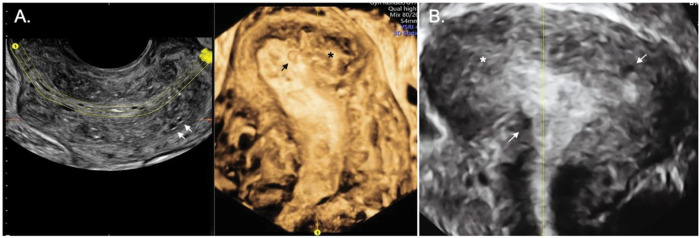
Two and three-dimensional ultrasound imaging of a uterus with diffuse type adenomyosis. A. Heterogeneous uterus with an irregular and interrupted junctional zone associated with myometrial cysts (arrows). B. Severe diffuse adenomyosis. Globular, enlarged, and heterogeneous uterus with myometrial cysts and an ill-defined junctional zone, completely infiltrated by adenomyosis (arrows and asterisks).
